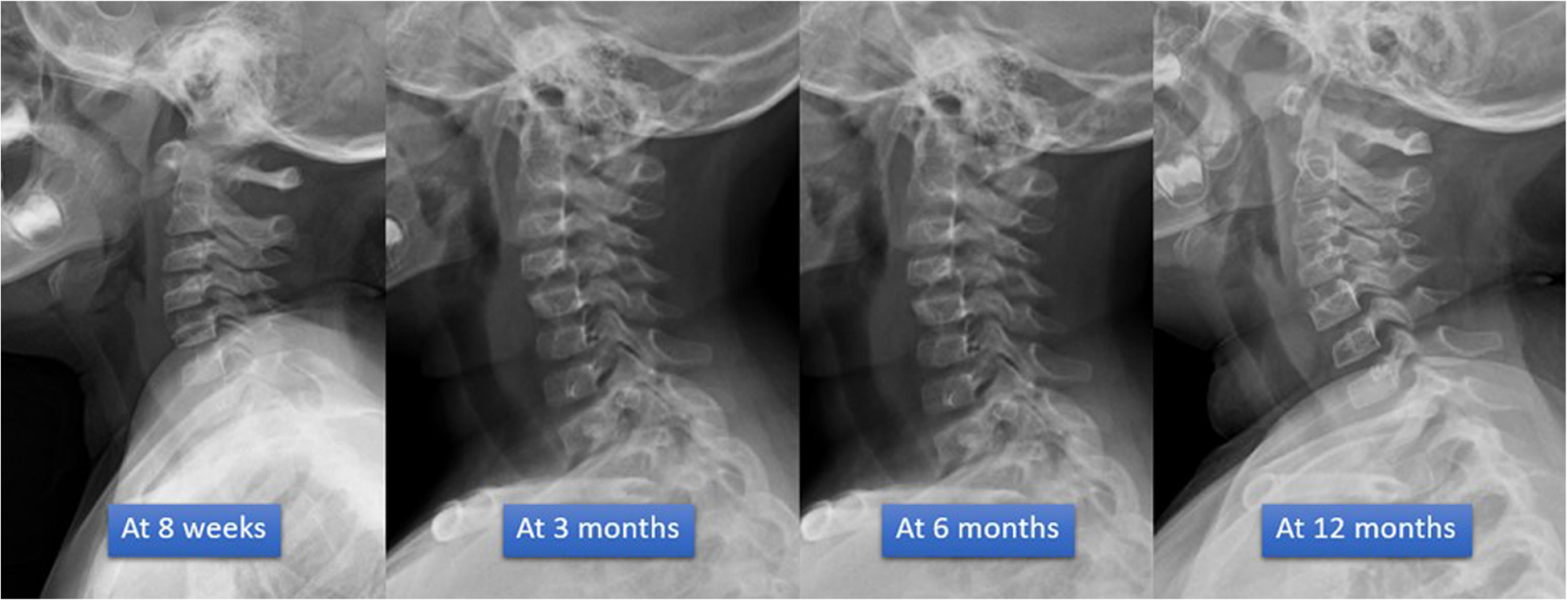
Radiographs images after removing minerva cast; showing the cervical spine had improved alignment and healed in follow up radiographs
